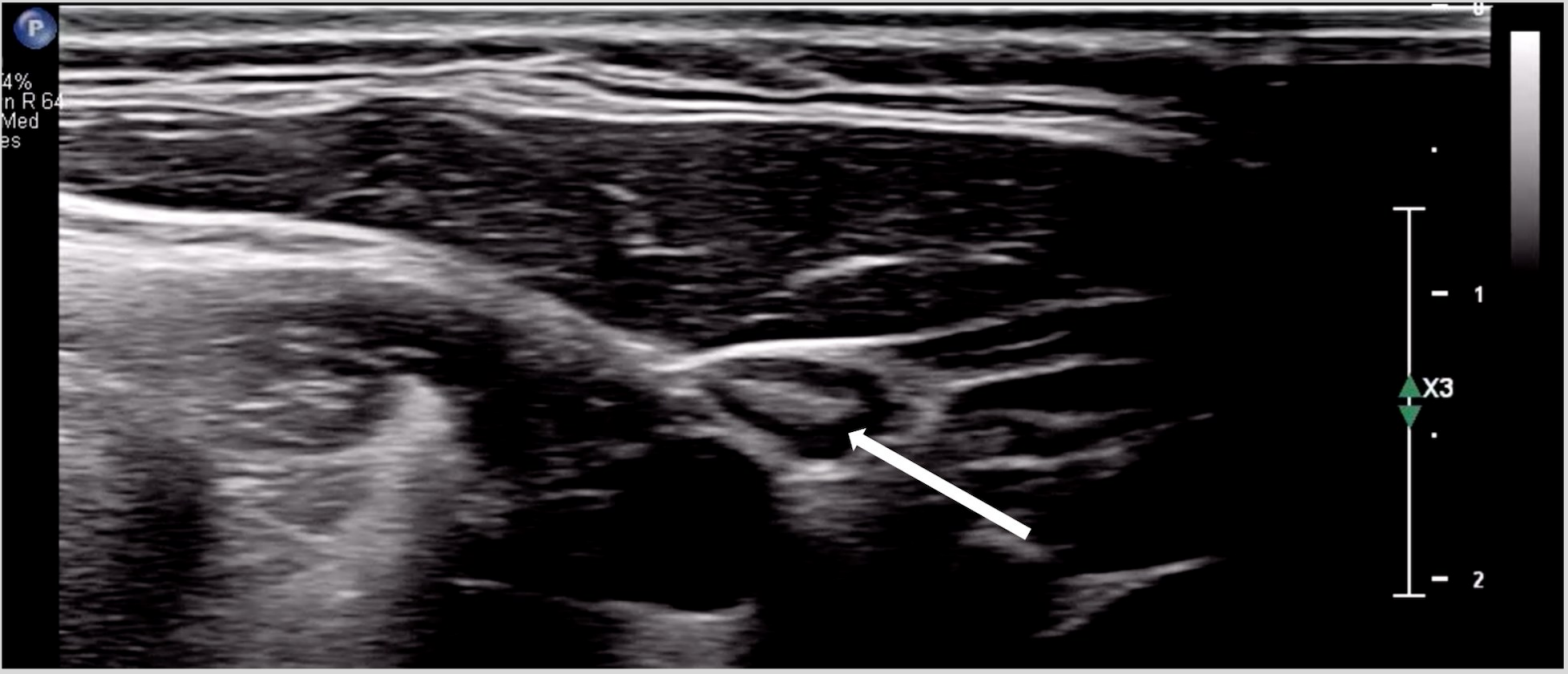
Point of care ultrasound (POCUS) of normal Lymph node (arrow indicating presence of hilum).
